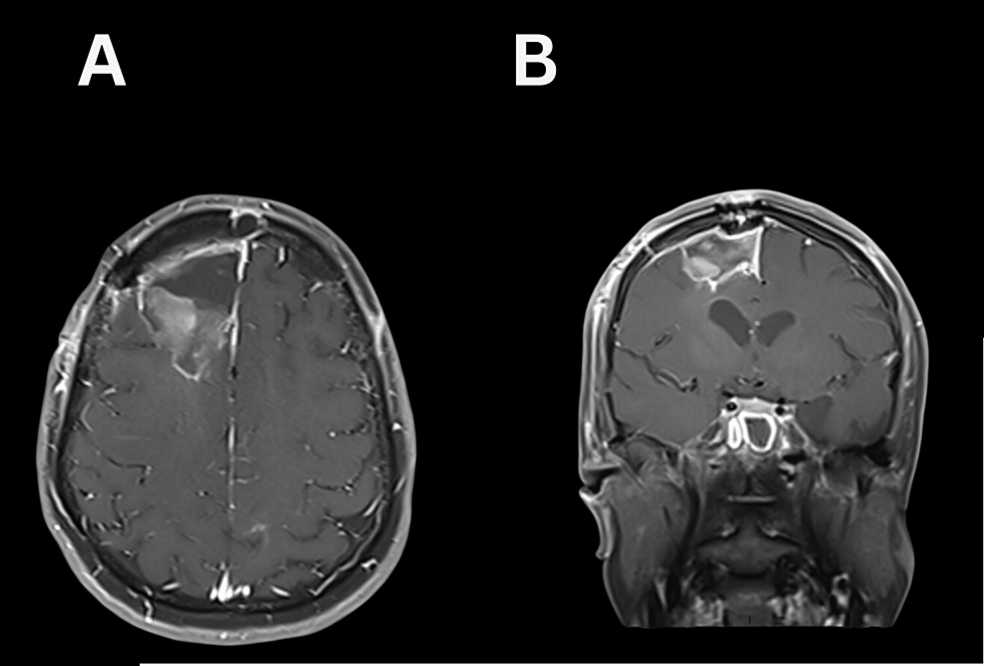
Brain MRI following gross total resection (A: T1+Contrast Axial; B: T1+Contrast Coronal).
